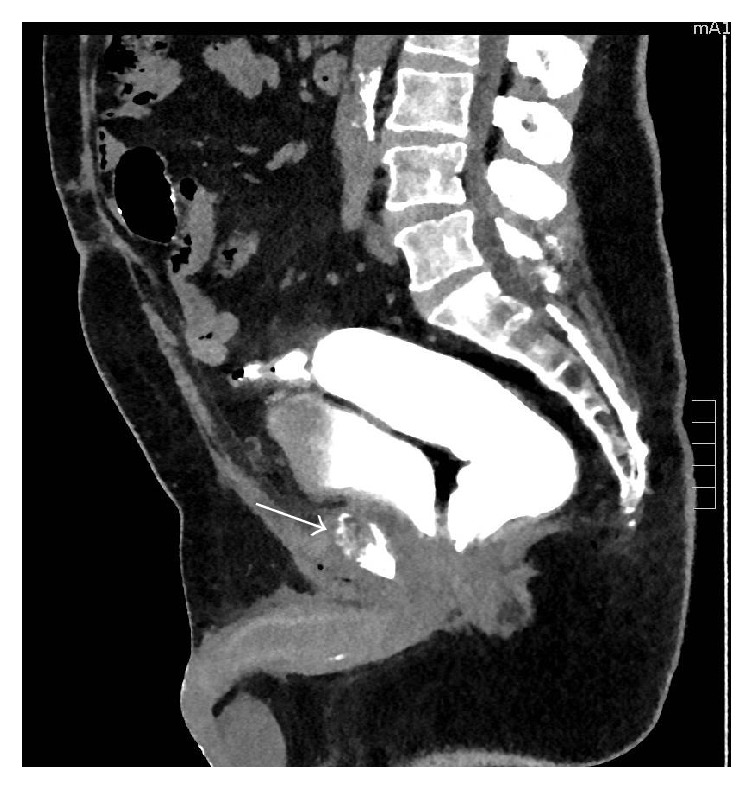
CT sagittal view of the abdomen and pelvis after administration of rectal enema, following the retrograde urethrogram. No evidence of contrast leakage from the bladder, urethra, or rectum is seen. No air bubbles are seen in the retropubic area in the location of the suture material that was reported on the previous MRI, confirming that the appearance on the MRI was related to surgical sutures, rather than leak. The bony abnormality in the symphysis pubis is also demonstrated in the view (arrow).
